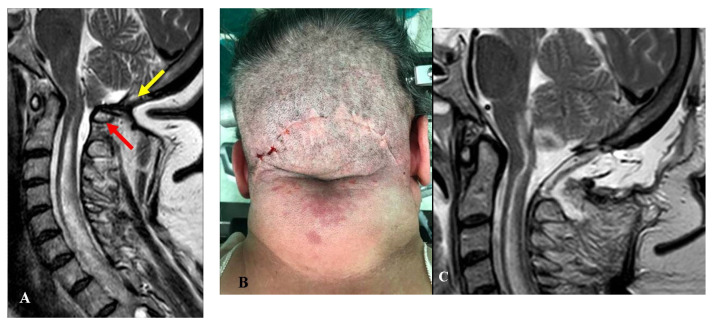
This 64-year-old woman had been treated with osteodural decompression, leaving C1 intact. One year later, she was referred to us for severe nocturnal breathing disturbances and mild quadriparesis. (A) Preoperative MRI, T2-weight, sagittal view, showing a small craniectomy with C1 still maintaining a significant obliteration of the arachnoid space (red arrow). A wide syringomyelia was evident. You will notice a marked skin retraction over the craniectomy (yellow arrow). (B) Intraoperative picture showing an “arc” incision and the skin retraction. The reoperation consisted of C1 laminectomy, tonsillectomy, and dural sac augmentation. (C) Postoperative MRI, T2-weight, sagittal view showing a relatively large subarachnoid space and initial syrinx shrinkage. There was mild local cerebellar edema owing to the tonsillectomy. An asymptomatic pseudomeningocele was also evident, which resolved spontaneously within a couple of weeks. Since the first postoperative period, breathing disturbances have significantly improved, whereas quadriparesis improvement has been slower. The final CCOS score was 10.
